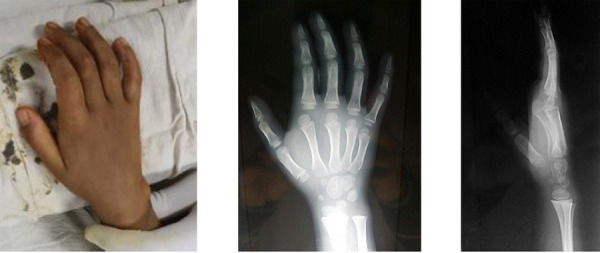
A) Pre-operative clinical picture, B) Preoperative X-ray images.
